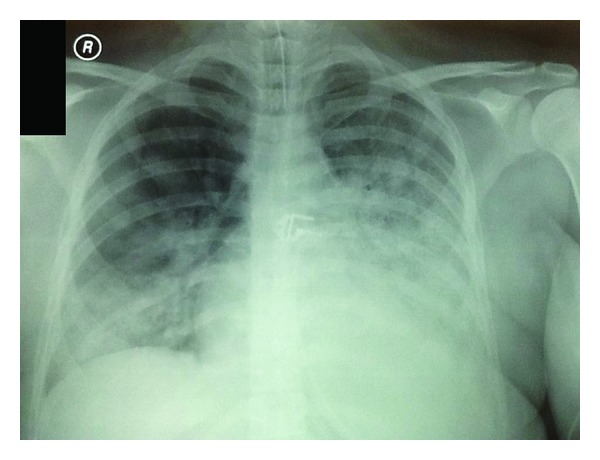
Chest radiograph of day 1 showing bilateral alveolar infiltrates and a dense area of consolidation with a small pleural reaction in the left hilar region.
